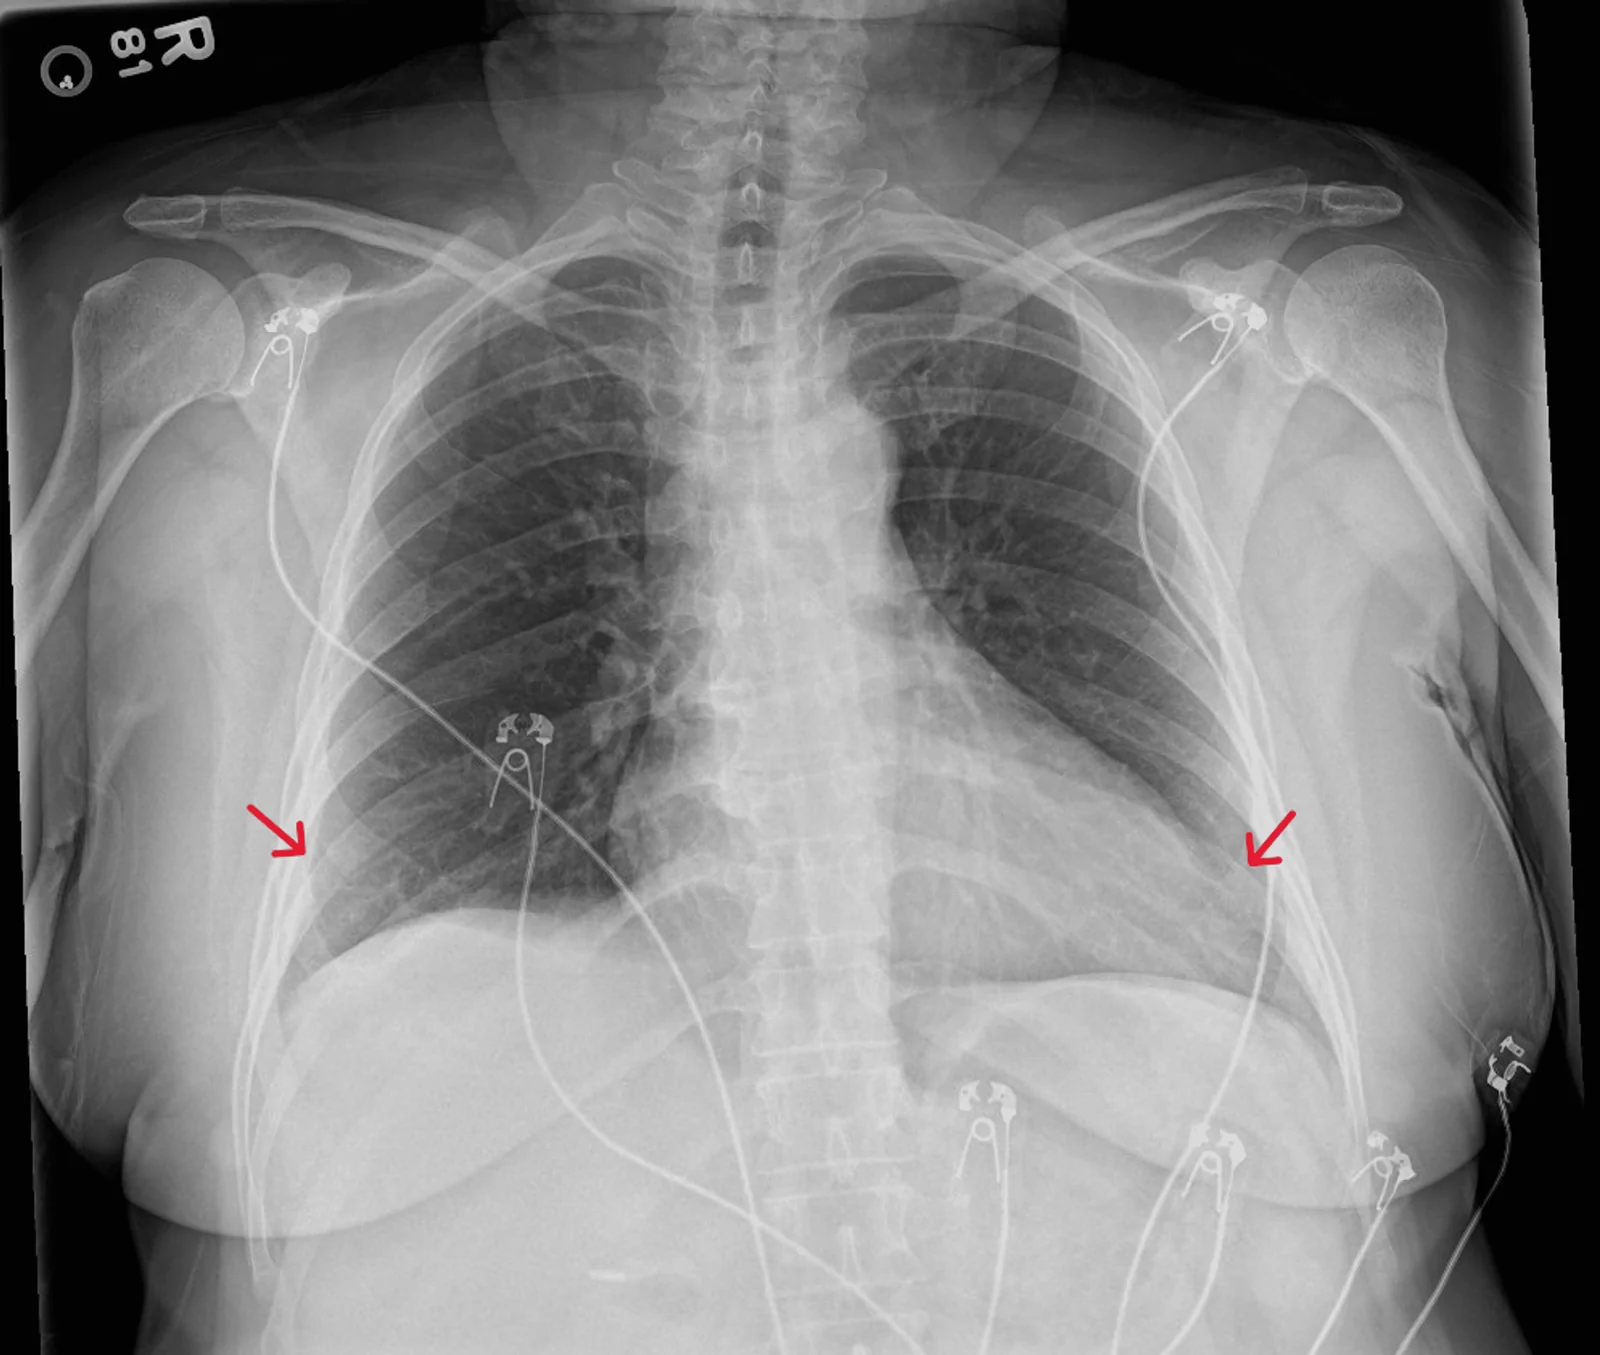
Repeat chest radiograph obtained during the patient's second emergency department presentation, demonstrating no acute cardiopulmonary abnormality. Red arrows indicate the regions of the previously identified bibasilar opacities, which had resolved, with no focal air-space consolidation or other acute cardiopulmonary abnormality.
